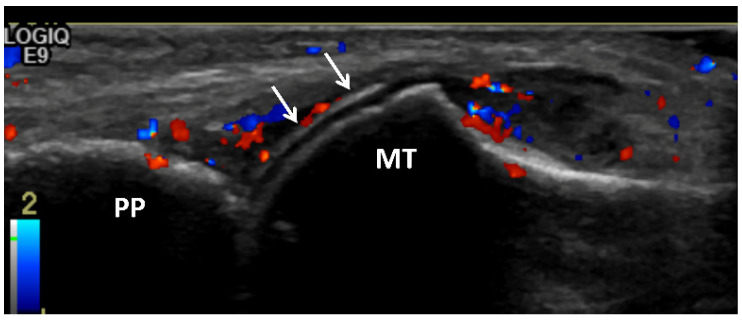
Gouty arthritis of the 1st metatarsophalangeal joint of 45-year-old male. Long-axis color Doppler US image along the dorsal aspect of the first metatarsophalangeal joint shows moderate distension of the joint capsule with moderate hyperemia consistent with synovitis. Note hyperechoic line that parallels the hyperechoic line of the subchondral bone, separated by anechoic cartilage along the metacarpal head (arrow) producing a “double contour” sign related to MSU crystal deposition. MT = metatarsal head. PP = proximal phalanx.
